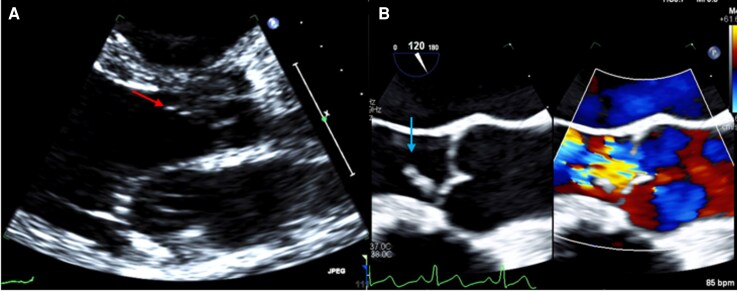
Echocardiographic findings in aortic valve endocarditis. (*A*) Initial transthoracic echocardiography (parasternal long-axis view) demonstrating a possible echogenic lesion on the aortic valve suggestive of vegetation (arrow). (*B*) Transoesophageal echocardiography (mid-oesophageal long-axis view) demonstrating a mobile aortic valve vegetation (arrow) with associated colour Doppler evidence of aortic regurgitation.
